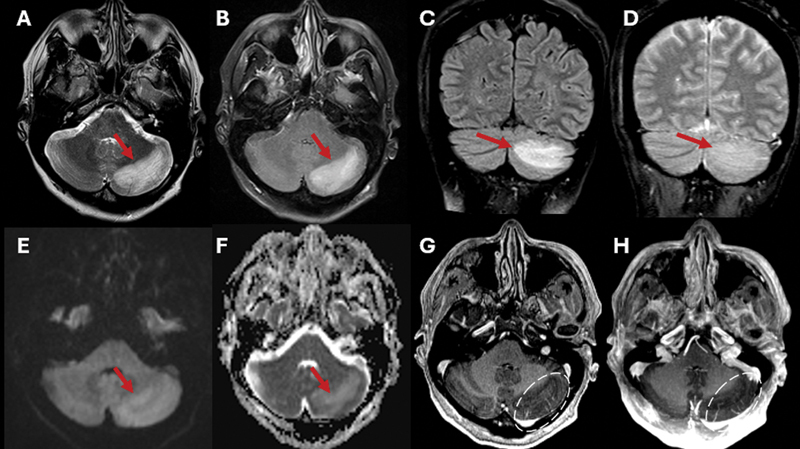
Initial brain magnetic resonance imaging (MRI) scan. (
